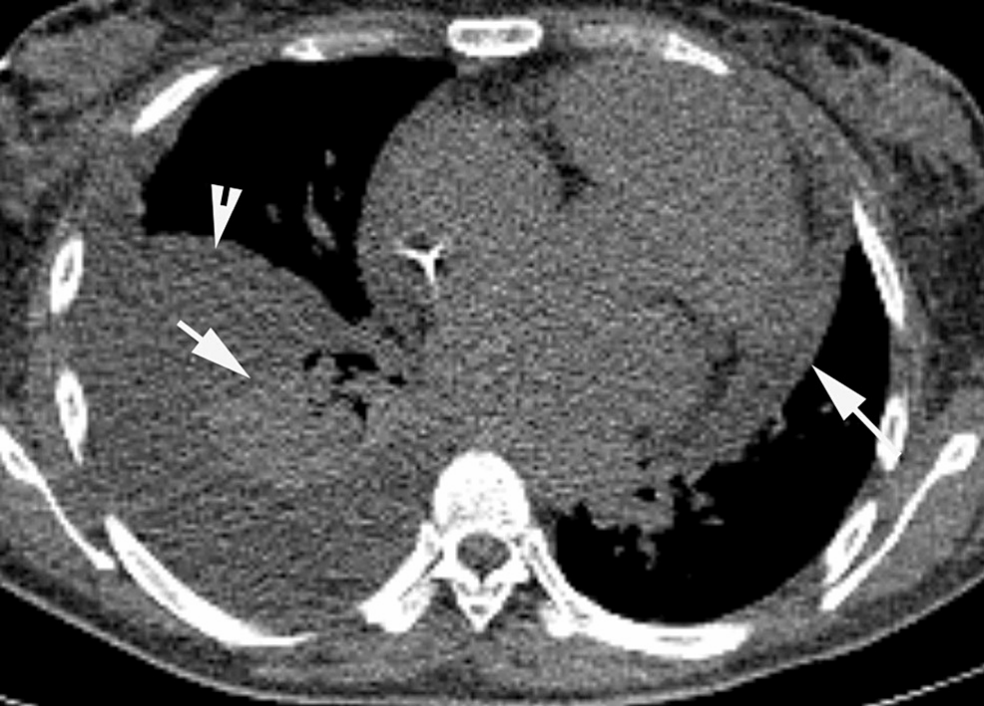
Non-contrast enhanced chest CT Incidental findings on CT images obtained for attenuation correction: pericardial effusion (long arrow), right lung atelectasis (short arrow), and right side pleural effusion (arrowhead)
